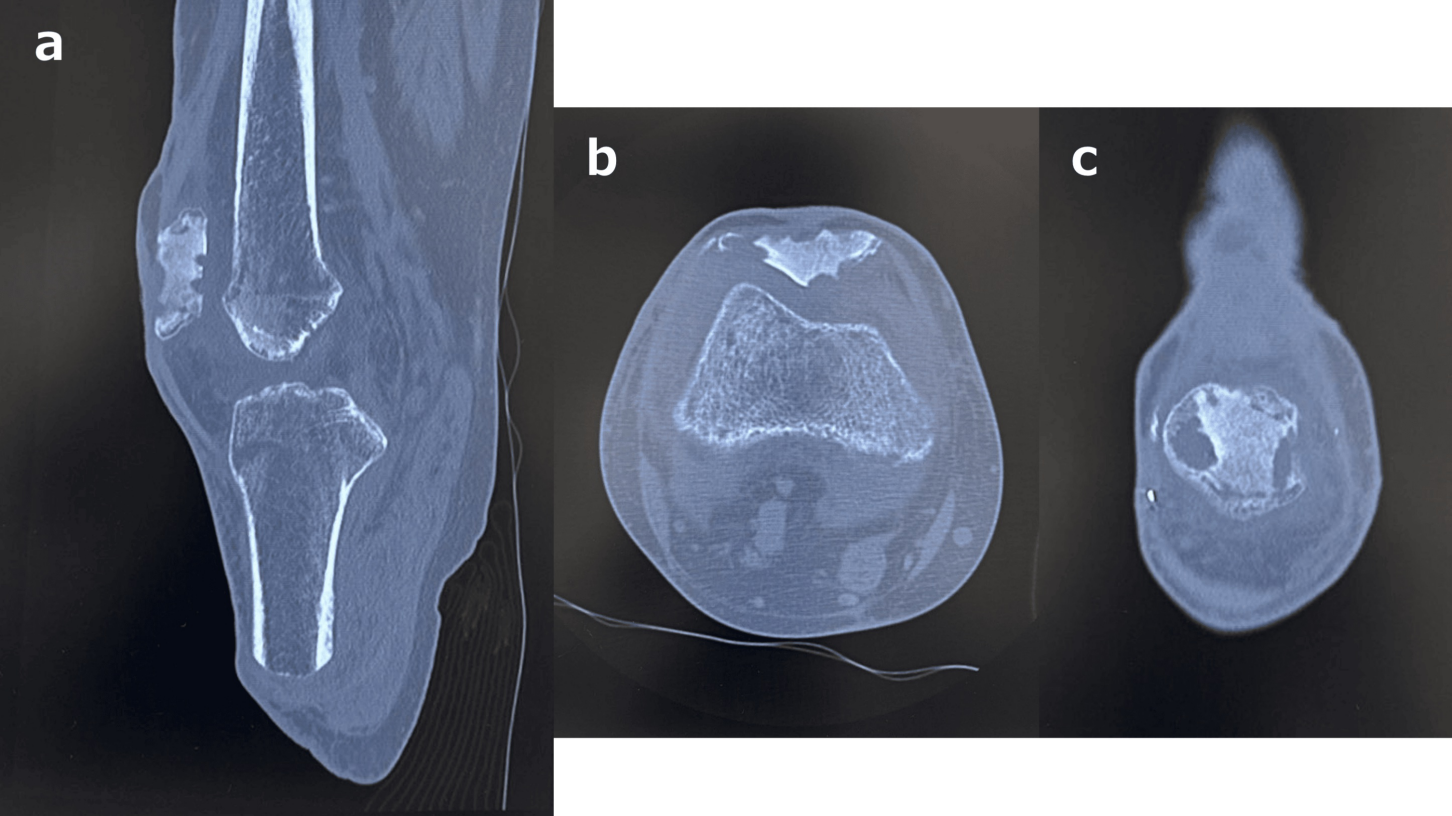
Computed tomography images of the osteomyelitis of the right patella. (a) Sagittal plane; (b) axial plane; (c) coronal plane.
